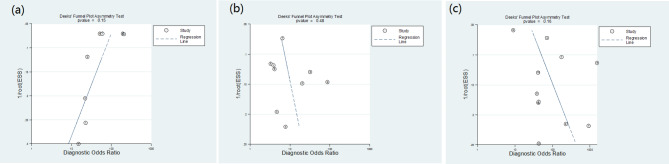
Funnel plot of ^18^ F-PSMA-1007 PET/CT (a), ^18^ F-FDG PET/CT (b) and ^18^Ga-PSMA PET/CT (c)
